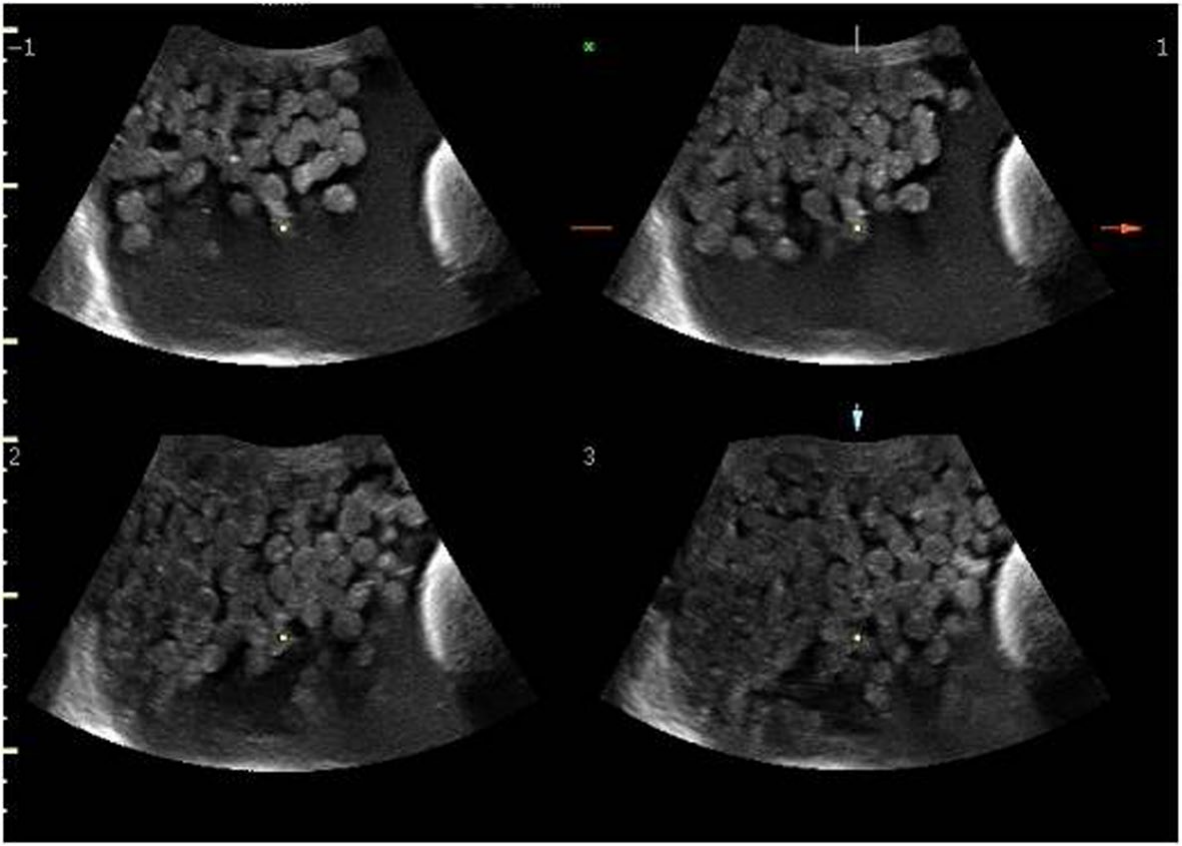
Ultrasound examination shows the extreme mobility of the endocystic formations to the movements impressed by the probe
